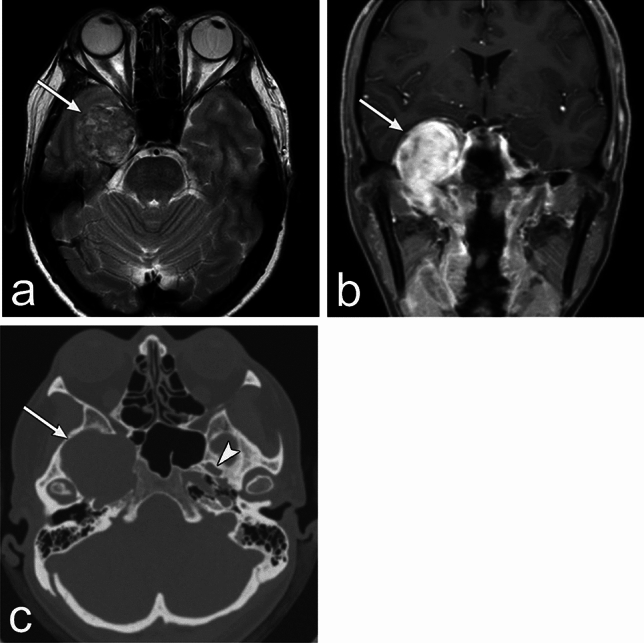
Trigeminal schwannoma of an asymptomatic woman in her 50s. The lesion is identified incidentally. a, b MRI shows a well-defined mass (arrow) in the right Meckel’s cave and right foramen ovale with a fusiform appearance along the nerve. The mass shows heterogeneous signal intensity with an equal to high signal compared to the brain parenchyma on T2 weighted image (WI). The mass shows heterogeneous enhancement. c CT shows dilatation of the right foramen ovale (arrow). The arrowhead indicates a normal left foramen ovale
